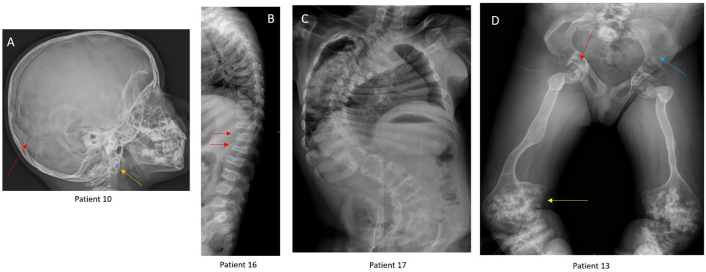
A. Radiographic features of SERPINF1 cohort. Patient (ID 10) lateral radiograph of skull in a four-year-old shows the Wormian bones (red arrow) and basilar invagination (yellow arrow), B. spine radiograph of a 2 year old patient (ID 16) shows multiple vertebral compression fractures, C. A 15 year old patient (ID 17) shows scoliosis, D. a 16-year-old (ID 13) patient AP view of pelvis and both femur shows protrusio acetabuli (red arrow), popcorn calcification in the epiphysis (yellow arrow) and lytic lesions (blue arrow) in the pelvic bones.
